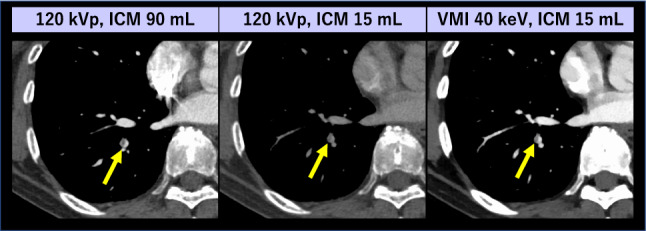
A 68-year-old woman with ovarian cancer and pulmonary embolism underwent two CTPA scans. The initial CTPA (left) was performed with a standard protocol (ICM 90 mL [370 mgI/mL]) using single-energy CT scanner. The follow-up CTPA (middle and right) was performed with a reduced ICM protocol (ICM 15 mL [370 mgI/mL]) using dual-layer detector scanner. In lower ICM dose protocol, a small pulmonary embolism (arrows) is barely visible at 120 kVp but easily detectable at VMI 40 keV, similar to the visibility in a standard ICM dose 120-kVp image
